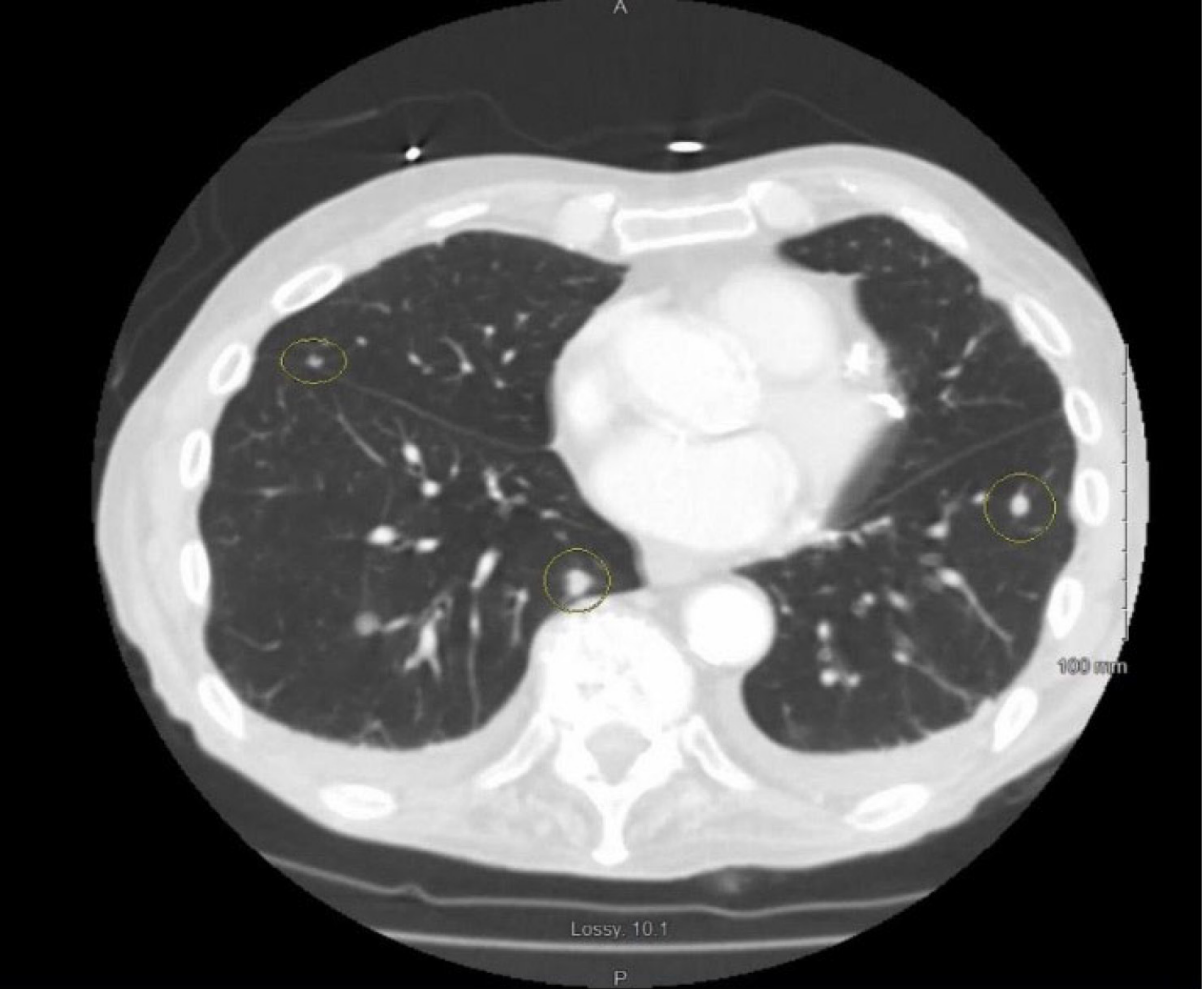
CT chest demonstrating bilateral noncalcified pulmonary parenchymal nodules (circled).
